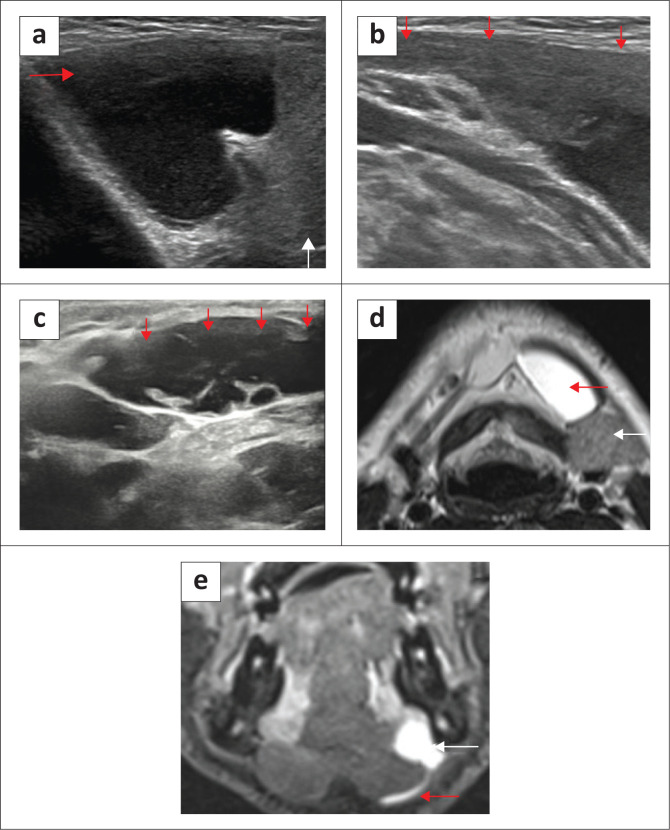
A case of a ranula in a 9-year-old child with a painless submandibular swelling. Ultrasound (a, b) showed a sonolucent cystic mass (4 cm × 2 cm; red arrows) with homogeneous low-level internal echoes, located medial to the submandibular gland (white arrow) and dissecting into the floor of the mouth. A different case of a ranula in an adult patient. Ultrasound (c) demonstrated a cystic mass, with internal debris and septations, in the left anterior submandibular region. MRI indicated the ranula (d, red arrow) on the T2W axial (d) and confirms extension to the sublingual space (e, white arrow) and communicating channel (e, red arrow).
